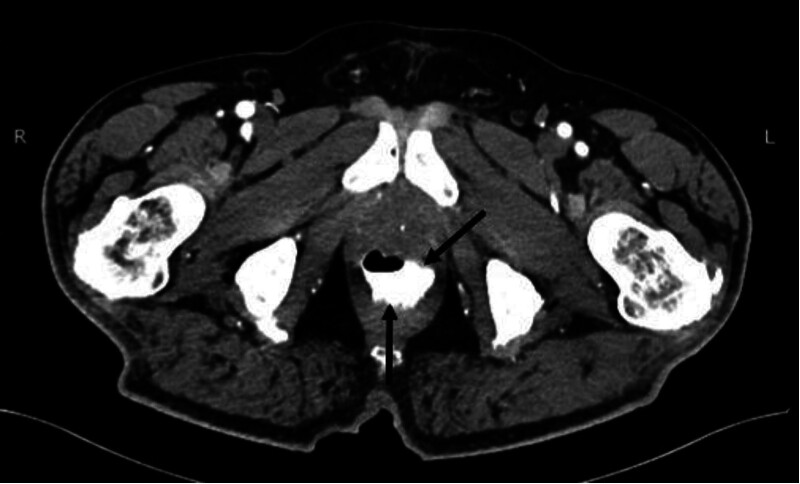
Follow-up CT scan taken 7 weeks postoperatively showing complete resolution of the rectal hematoma, shown in Figure 3. (black arrows). CT = computed tomography.
